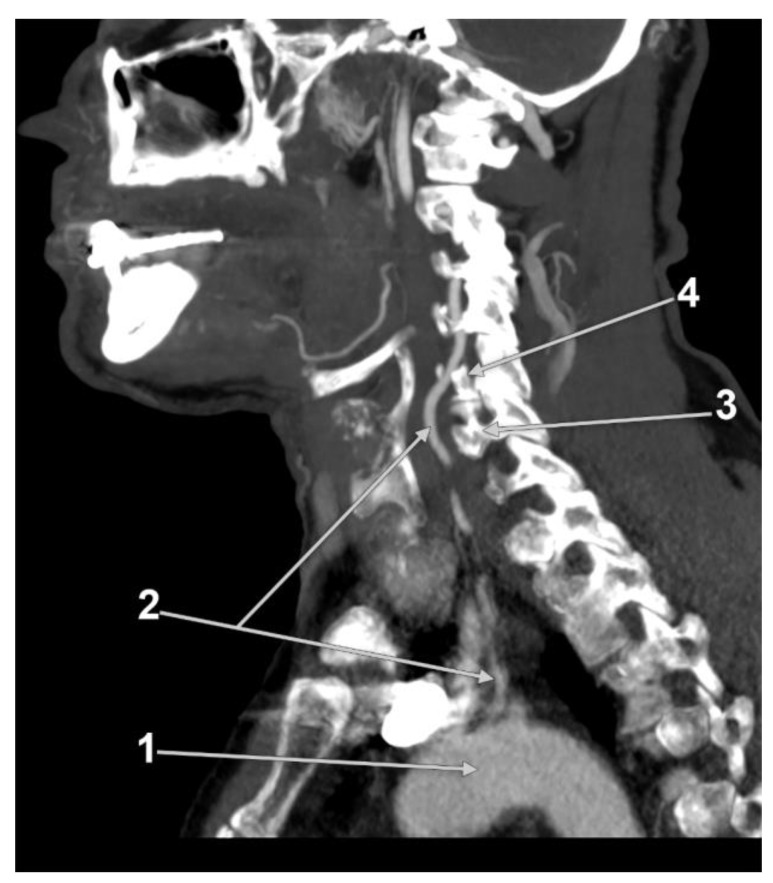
Computed tomography angiogram. Sagittal slice through the left vertebral artery originating the aortic arch and entering the transverse foramen of the fifth cervical vertebra. Original evidence: 1. aortic arch; 2. left vertebral artery; 3. transverse process of sixth cervical vertebra; 4. transverse process of fifth cervical vertebra.
